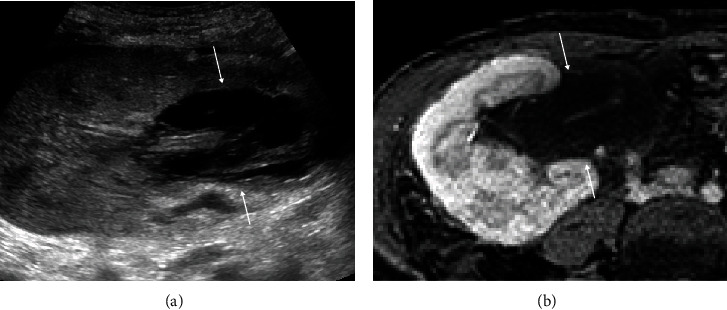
Right lower quadrant transplanted kidney. Ultrasound shows a complex, septated collection in the renal pelvis mimicking hydronephrosis (arrows, (a)) which was confirmed on the corresponding postcontrast fat-saturated axial MRI (arrows, (b)).
